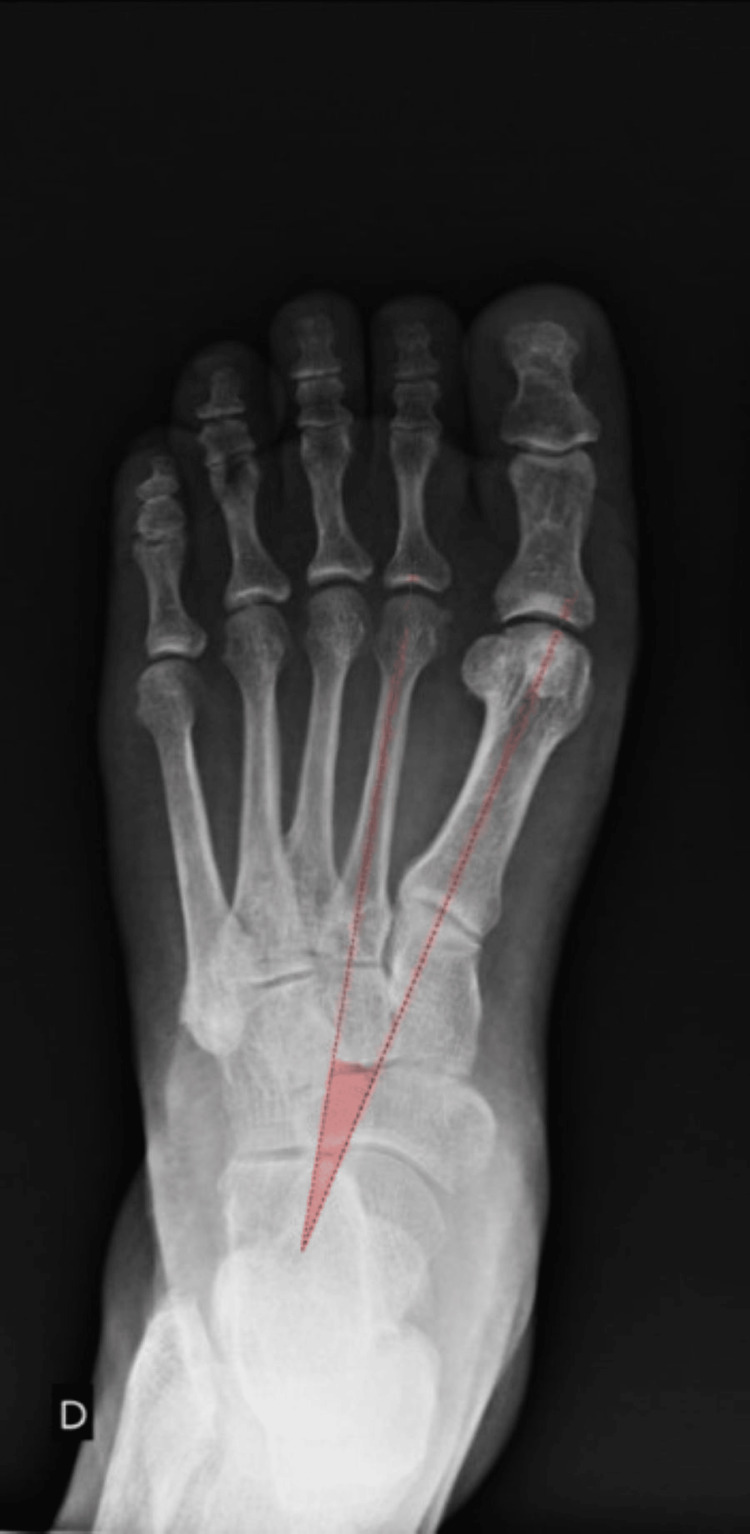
Anteroposterior (AP) foot radiograph demonstrating the measurement of the first–second intermetatarsal angle (IMA), with values less than 9° considered within the normal range. Courtesy of Leonardo Lustosa, Radiopaedia.org, rID: 91174. Adapted from Leonardo Lustosa, Radiopaedia.org, available under the CC BY 4.0 International license (https://creativecommons.org/licenses/by/4.0/) [32].
